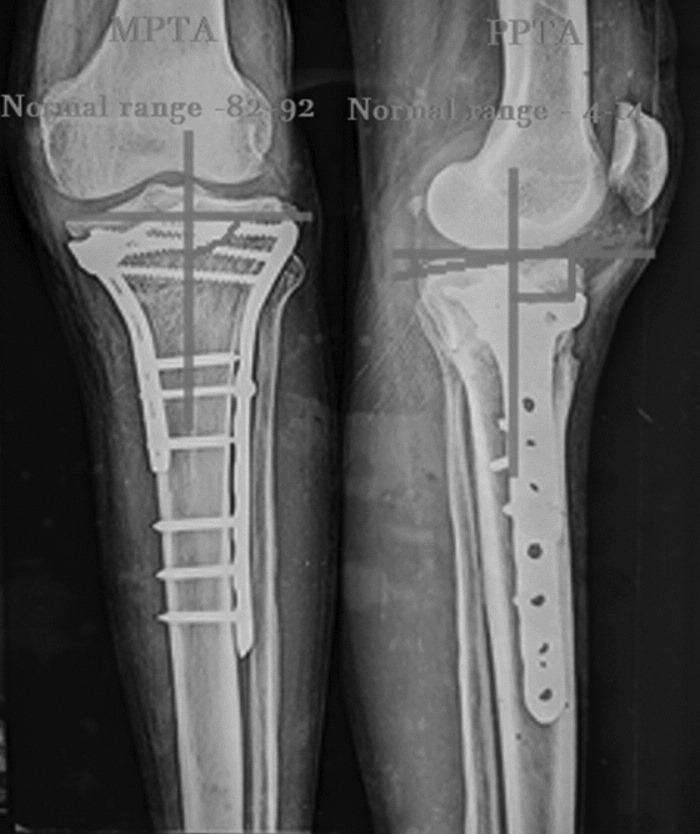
Radiographic measurements of MPTA and PPTA angle.
